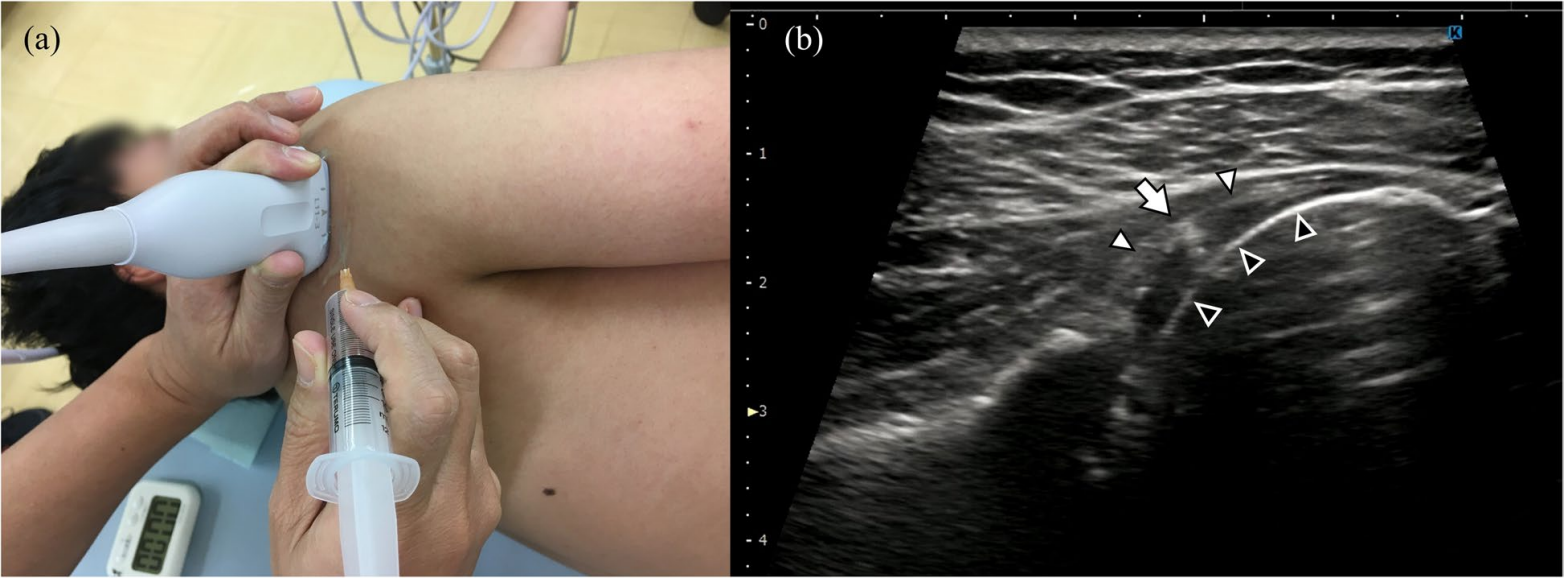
Glenohumeral joint injection. a With the patient in the half side-lying or lateral recumbent position, 5 mL of 1% mepivacaine and 20 mg of triamcinolone are injected into the glenohumeral joint under ultrasound guidance. b The white arrowhead is the capsule, the black arrowhead is the humeral head, and the white arrow is the needle tip
